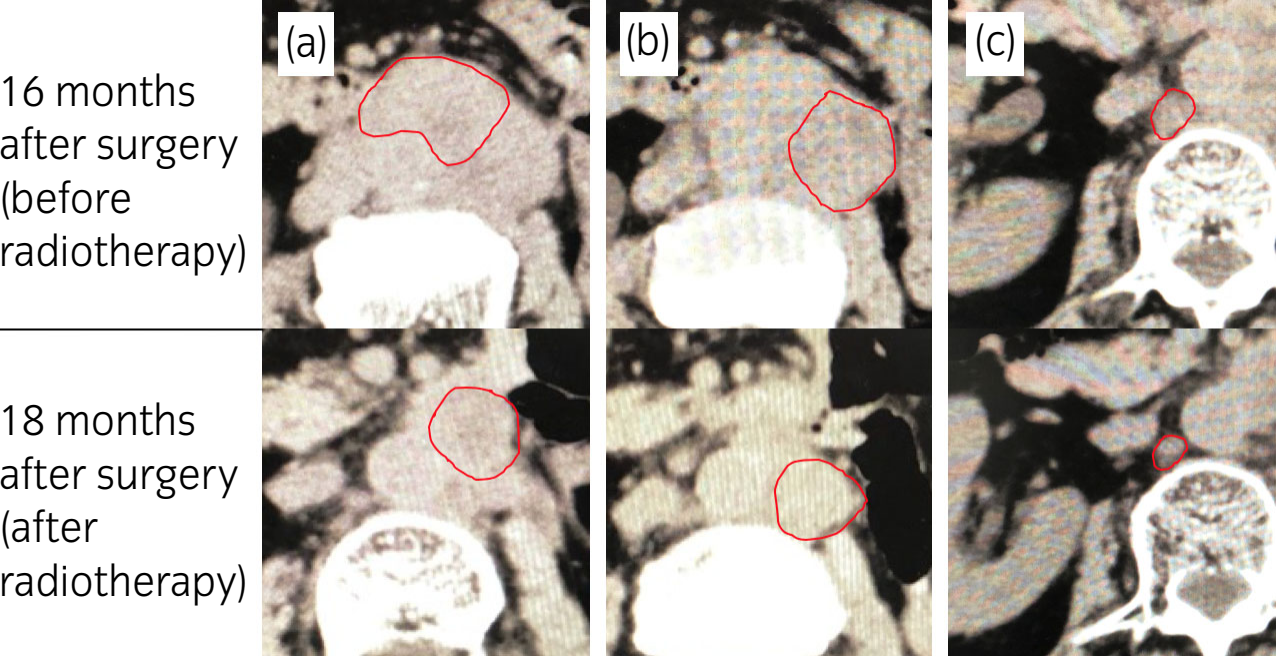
CT showing reduction in metastatic LNs after radiotherapy. (a,b) Paraaortic LNs. (c) Right renal hilum LN. The upper row shows LNs at 16 months after surgery (before radiotherapy); the lower row shows LNs at 18 months after surgery (after radiotherapy). The red line outlines the circumference of target lesions.
